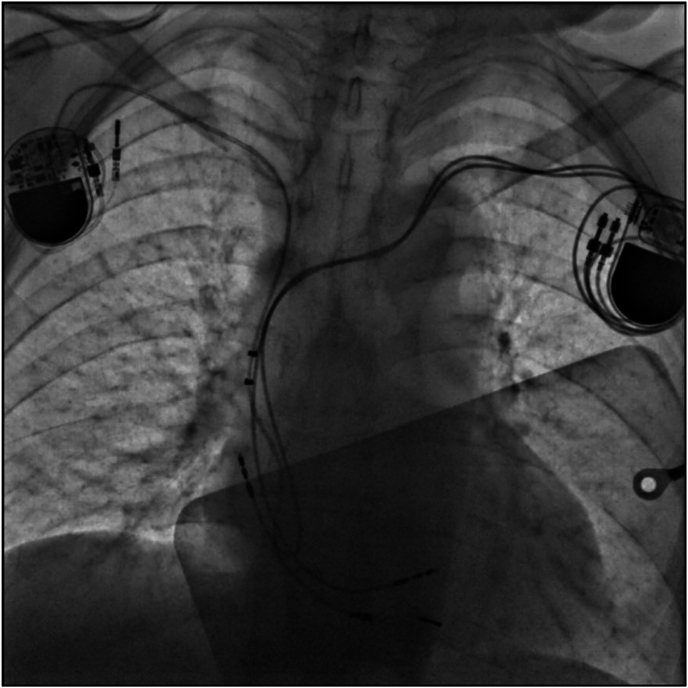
Chest X-Ray showing both devices.
